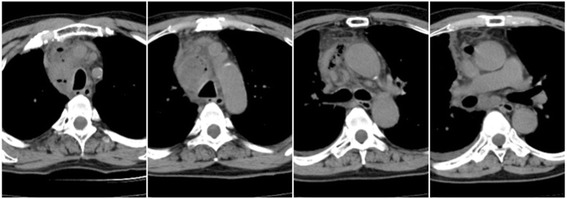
CT imaging of mediastinitis. Chest CT, performed 7 days after EBUS-TBNA, shows enlargement of the lower paratracheal lymph nodes and gas production in the mediastinum along with the appearance of right pleural effusion
